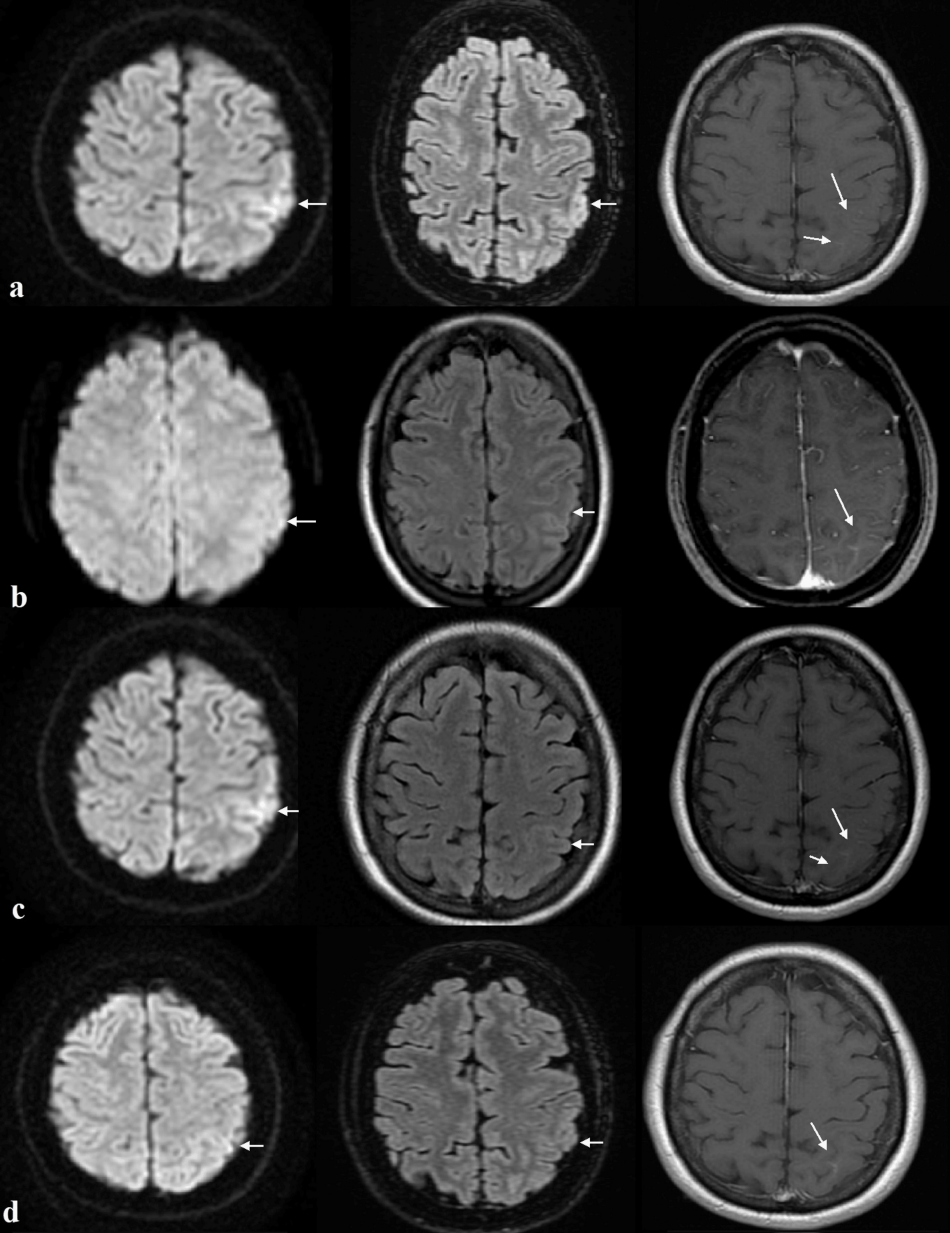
Axial MRI of the brain showing a high DWI and T2 signal unilateral cortical lesion at the left parietal lobe and leptomeningeal contrast enhancement (arrows). a. Performed nine days before her admission to our hospital;
